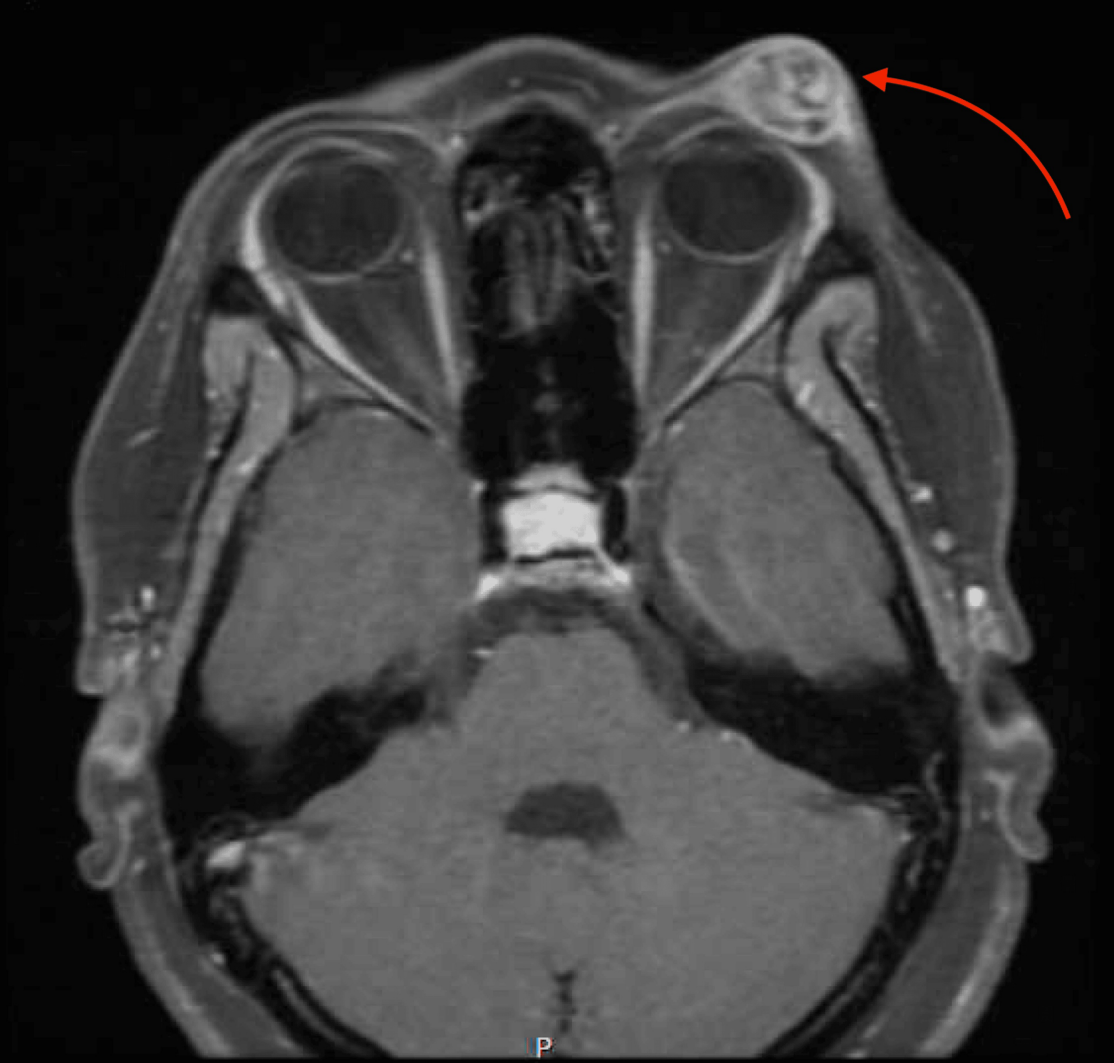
An axial T1 fat-saturated MRI of the orbit showing a large heterogeneous mass on the left eyelid MRI: magnetic resonance imaging
